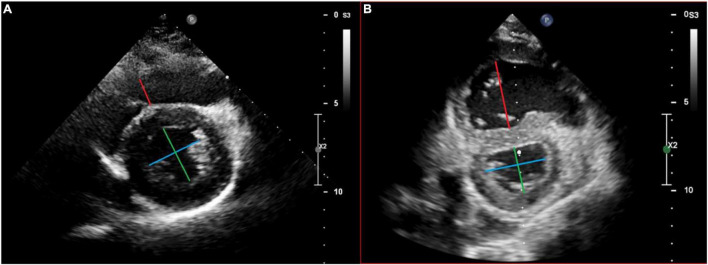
Echocardiographic measurement of the right ventricular to left the ventricular endsystolic (RV/LVes) ratio and the left ventricular endsystolic eccentricity index (LVes EI) in a healthy subject (A) and in a patient with pulmonary hypertension (B). Measurement of the RV/LVes ratio (RV = red/LV = green line) and the LVes EI (blue/green line) in the apical parasternal short axis view between papillary muscle, from the endocardial to endocardial surfaces at endsystole.
